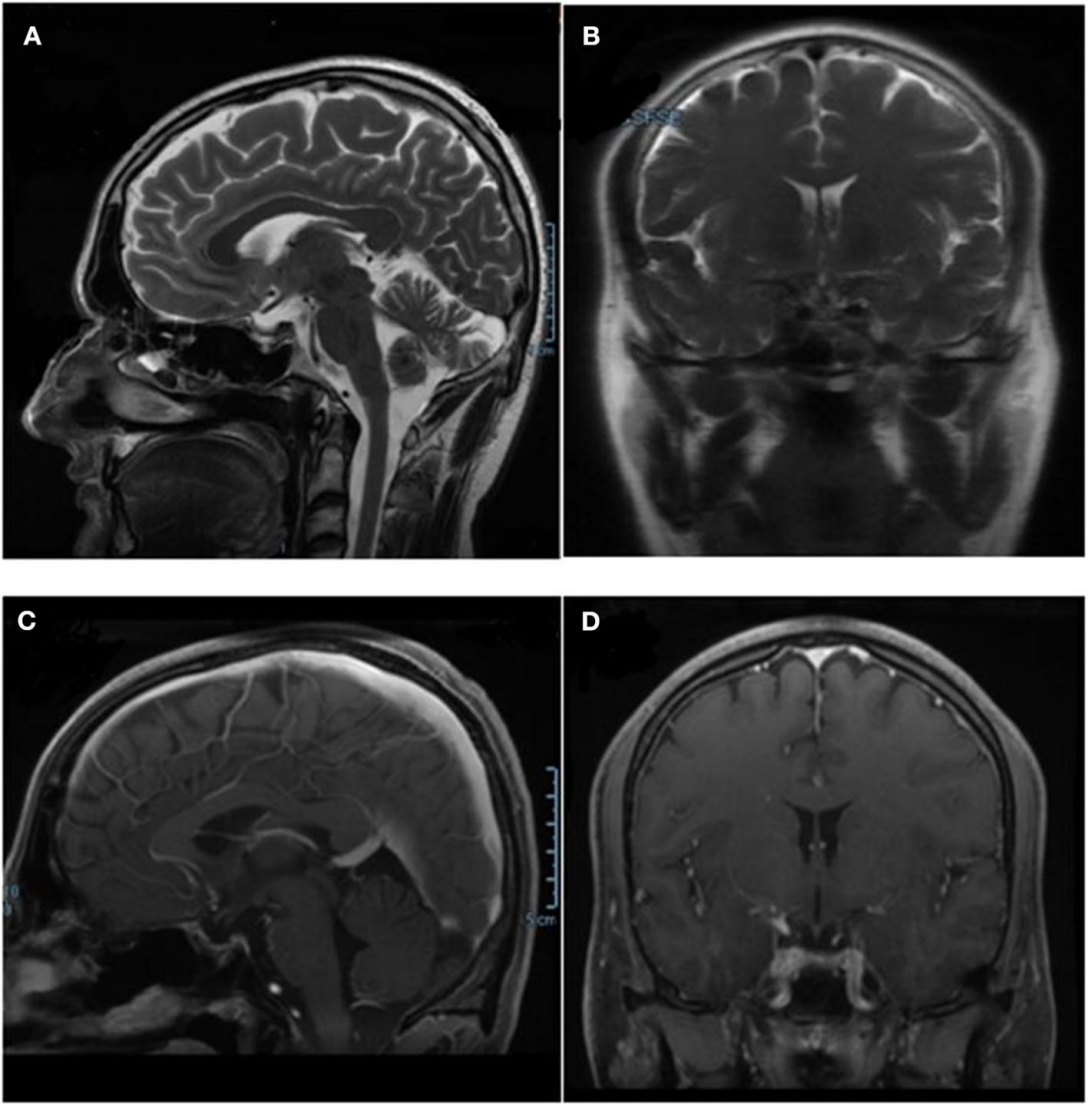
Pituitary MRI performed after combined therapy with dabrafenib and trametinib, showing the complete remission of the pituitary mass. (A,B) T2-weighted basal sagittal and coronal sequences. (C,D) T1- weighted post-gadolinium sagittal and coronal sequences MRI.
